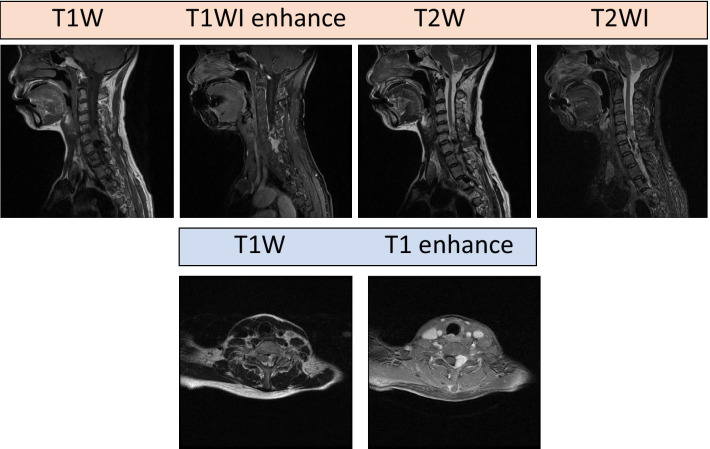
Magnetic resonance imaging of the patient. The left intervertebral foramen of C6-7, with an ill-defined margin that is 2.8 × 1.5 × 1.4 cm in size, and a slightly hyperintense T2WI signal like T1WI in the surrounding small portion, low T1WI and low T2WI signal without enhancement in the center. The spinal cord is compressed and pushed to the right. *T1* weighted imaging, T1WI; *T2* weighted imaging
